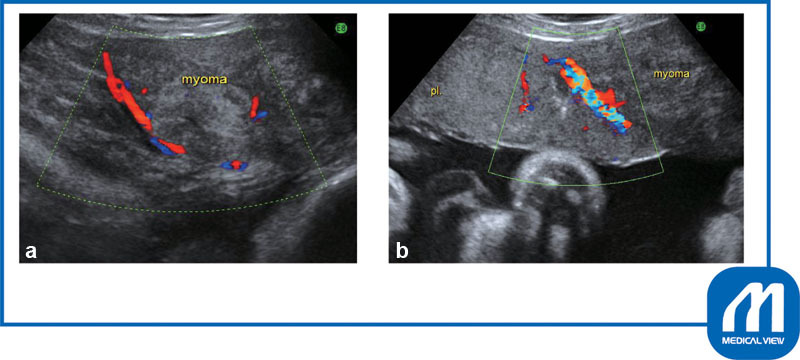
Case 1: Ultrasonography findings.
